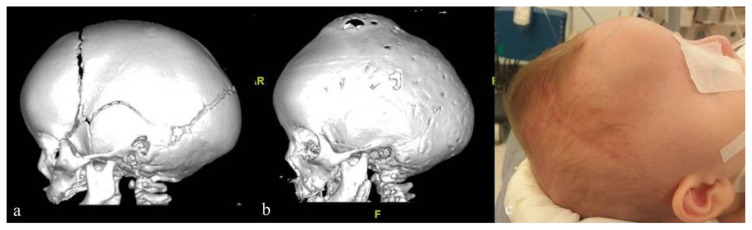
Case 6: 6 months old scaphocephaly patient. a: Image of first preoperative 3D CT, b: Image of second preoperative 3D CT, c: Photography before the second surgery shows bony bulging of vertex.
